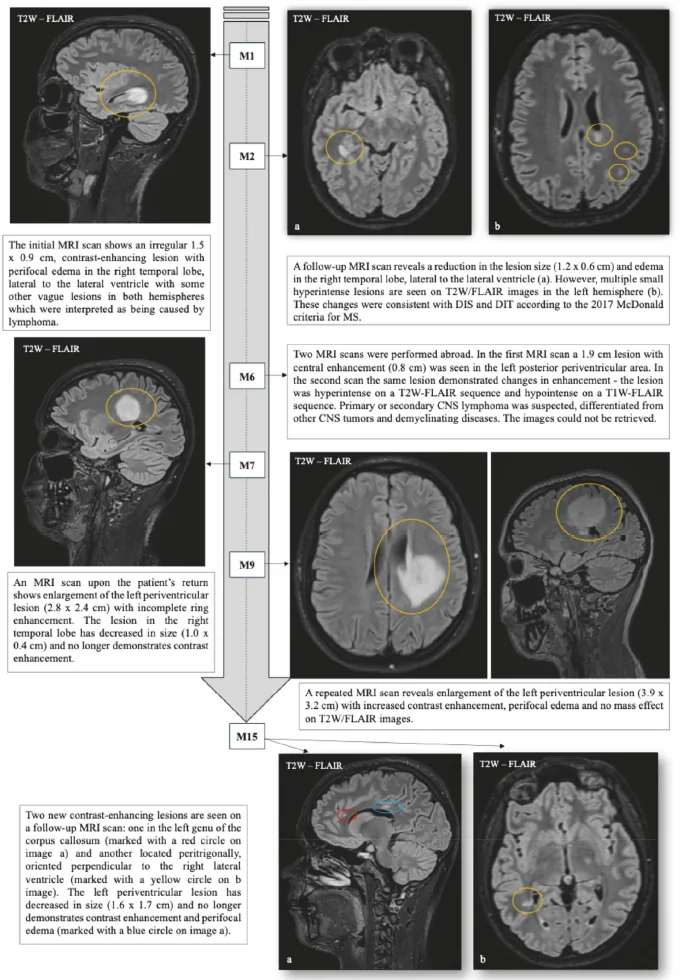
Graphical representation of magnetic resonance imaging changes from the onset of symptoms reported until the diagnosis. The letter ‘M’ followed by a number represents the month when the scan was performed. Magnetic resonance imaging sequences are specified in the upper left corner of each scan. Circles indicate the specific pathological feature discussed in the corresponding text below each scan *Note*. The following abbreviations are used: T2W-FLAIR, T2-weighted Fluid-attenuated inversion recovery; MRI, magnetic resonance imaging; M, month; cm, centimeter.
